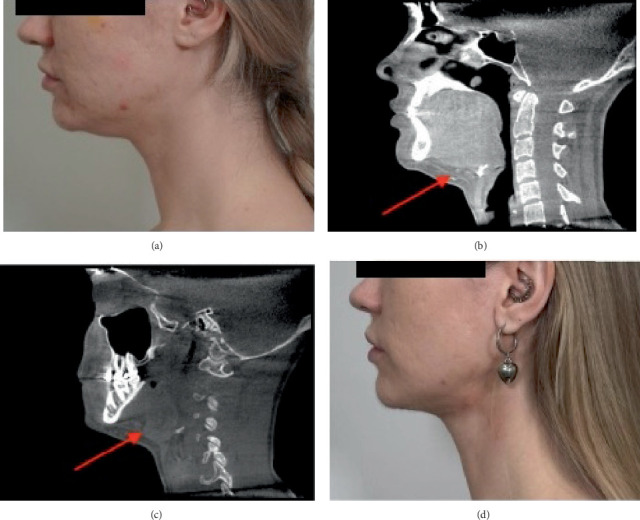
(a) Preoperative photograph showing submental fullness and blunted cervicomental angle. (b) Sagittal CBCT reconstruction demonstrating excessive accumulation of adipose tissue in the submental area, both subcutaneously and beneath the platysma muscle (red arrow). (c) CBCT image highlighting multiple high-density linear inclusions within the adipose tissue above the platysma (red arrow). (d) Postoperative photograph after surgical correction of the lower third of the face and neck.
